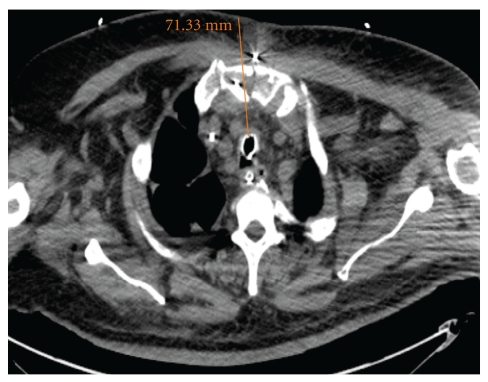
CT of an obese patient. Due to the distance of the trachea from the anterior surface of the neck (>7 cm from the skin), the trachea could be reached reasonably easily and safely from a point under the sternum.
